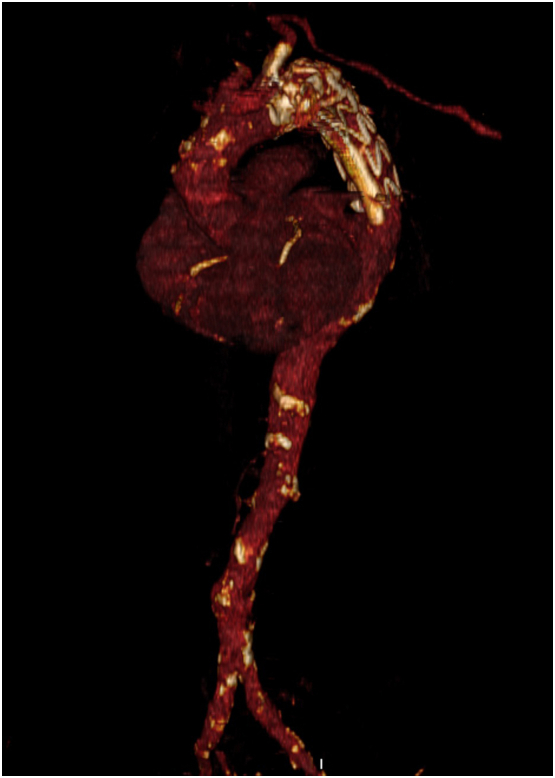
Postoperative CT Angiography 3-dimensional volume rendering showing TEVAR plus periscope graft and patency of left subclavian artery.
